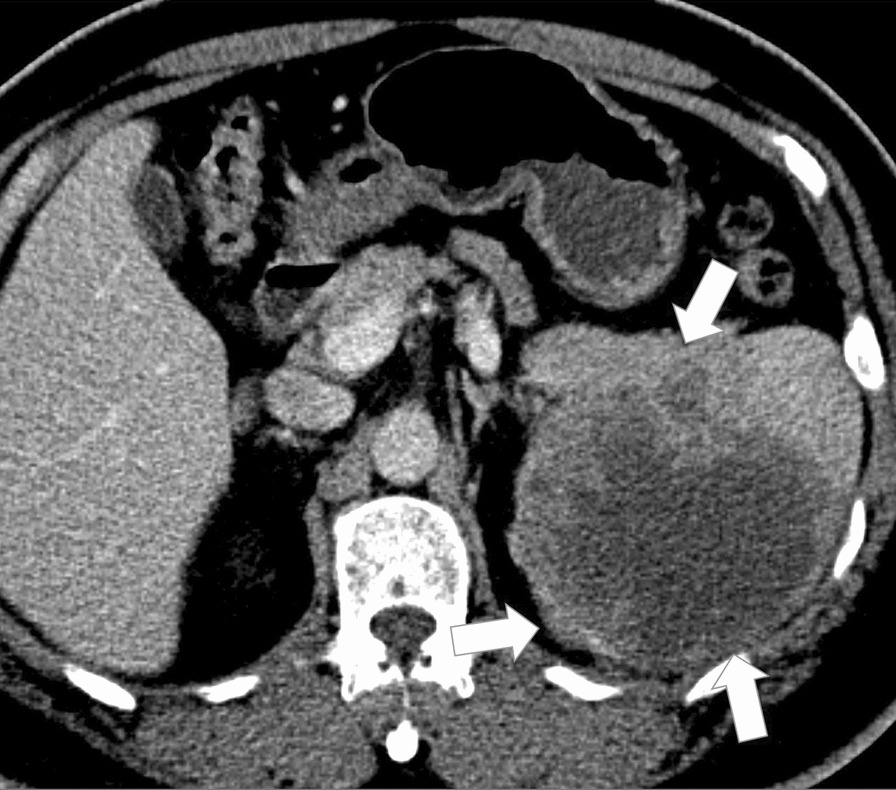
Contrast-enhanced abdominal CT showing a large heterogeneous and hypodense mass (arrows) involving the pancreatic tail and spleen
